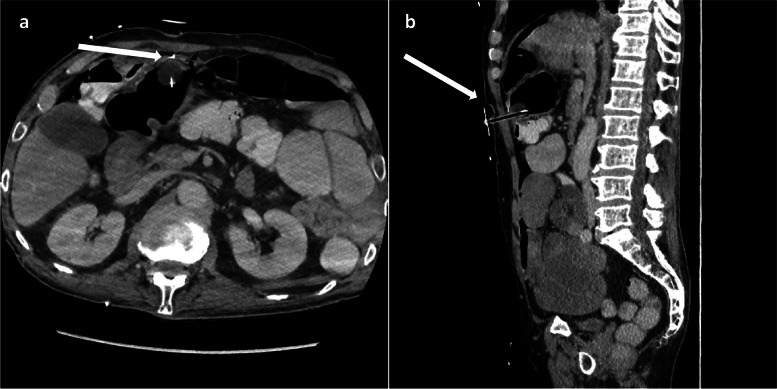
a, b Axial and sagittal CT images for patient who deteriorated on the ward post RIG insertion. White arrows show the gastrostomy tube traversing collapsed transverse colon
